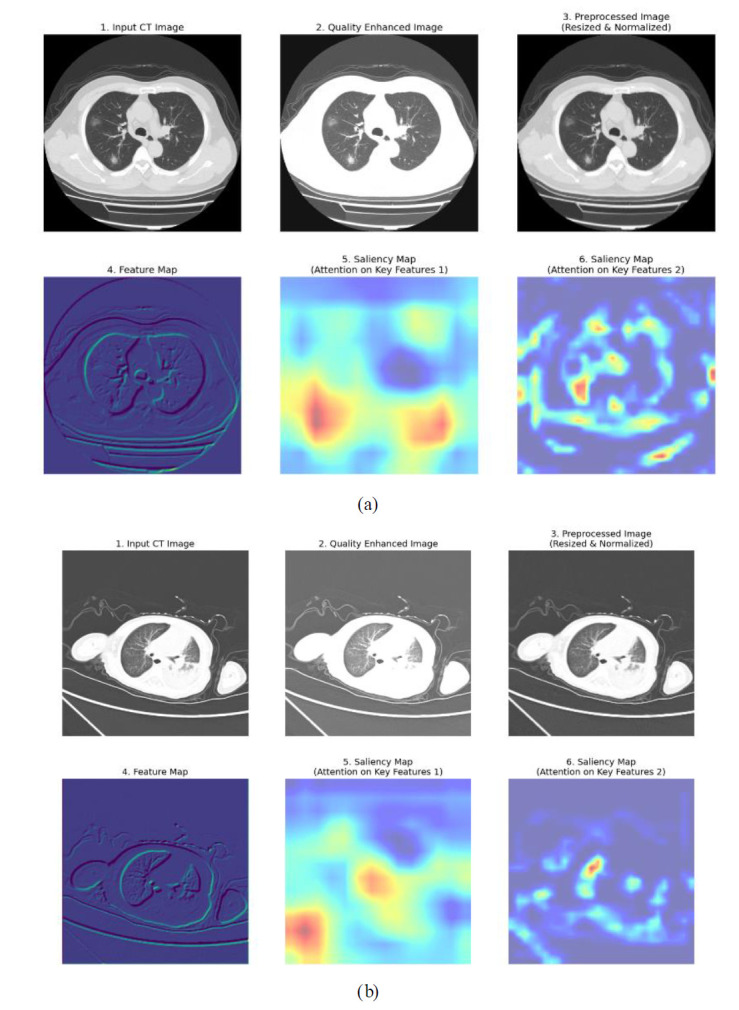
Sample input and output CT images.
